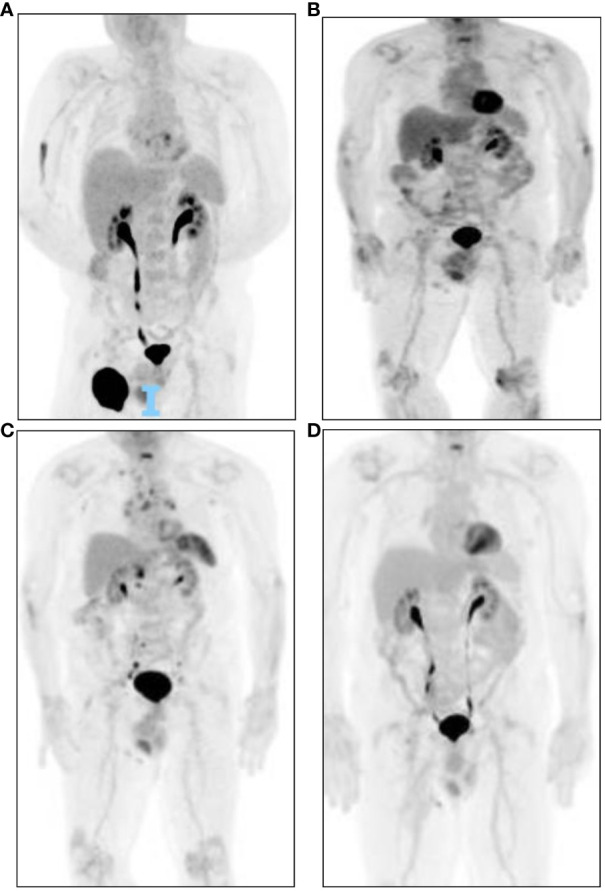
Case 2 PET progression. (A) Baseline PET scan on 30/05/2019 showing a large right inguinal mass and prominent subcarinal node with SUV max 2.9. (B) PET 28/08/2020, after completion of adjuvant therapy showing postsurgical changes in the right thigh and resolved FDG uptake of the subcarinal lymph node. (C) 21/07/2021 representative surveillance PET with mediastinal, abdominal, retroperitoneal, and right iliac lymph nodes (biopsy). (D) 28/06/2023, most recent PET. Postsurgical changes without abnormal lymphadenopathy. This patient received no sarcoid-directed therapy over this course.
